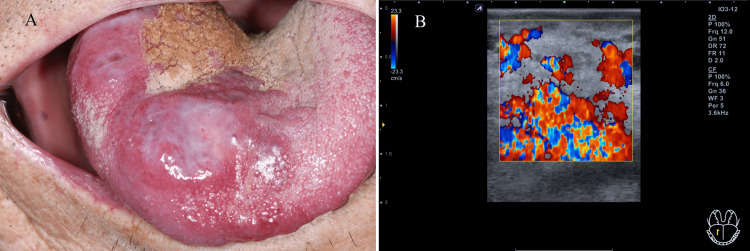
Vascular lesion on the anterior region of the tongue (A) Clinical appearance before treatment; (B) Ultrasonography image before treatment
